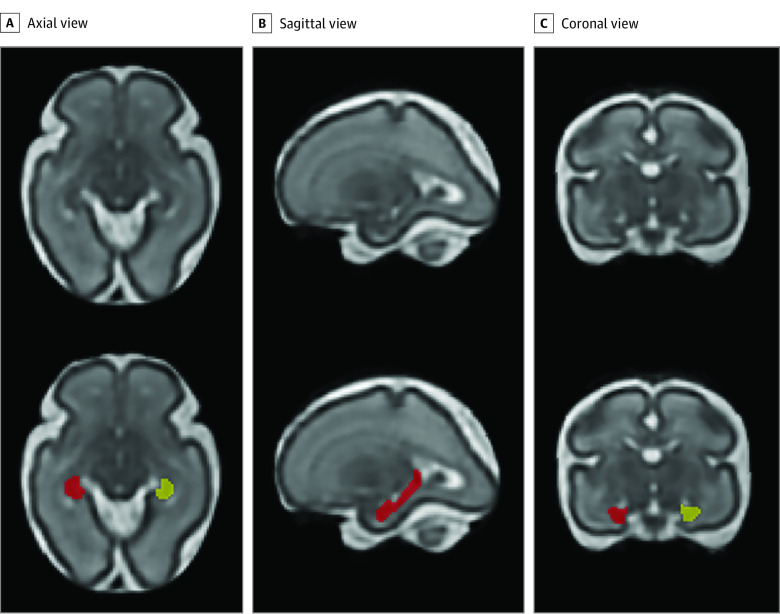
Segmentation of the Hippocampus Left hippocampus (yellow) and right hippocampus (red) are shown on a 3-dimensional reconstructed T2-weighted magnetic resonance image of a fetus at 27.9 gestational weeks.
